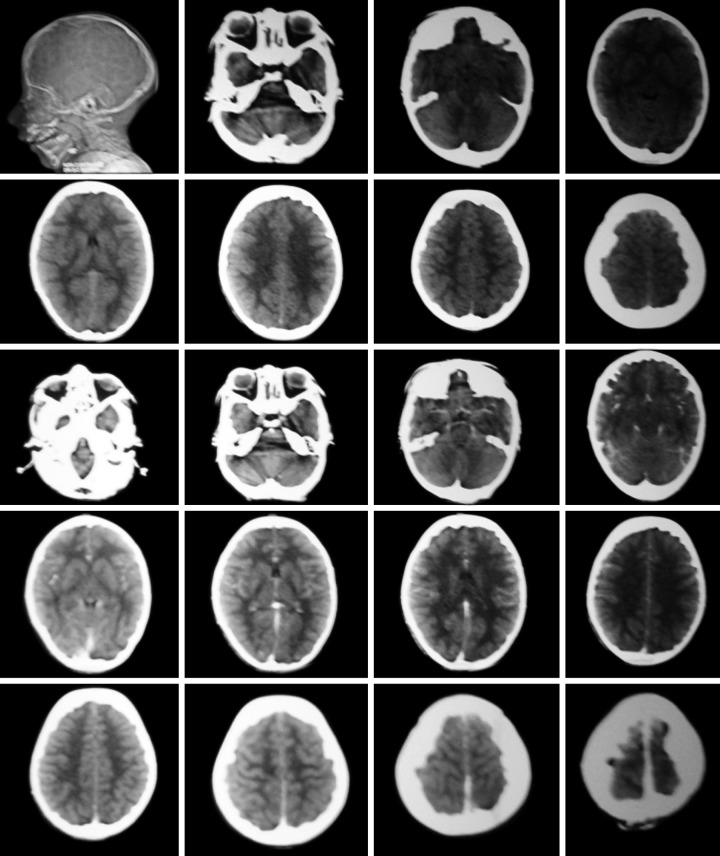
Computed tomography scan
